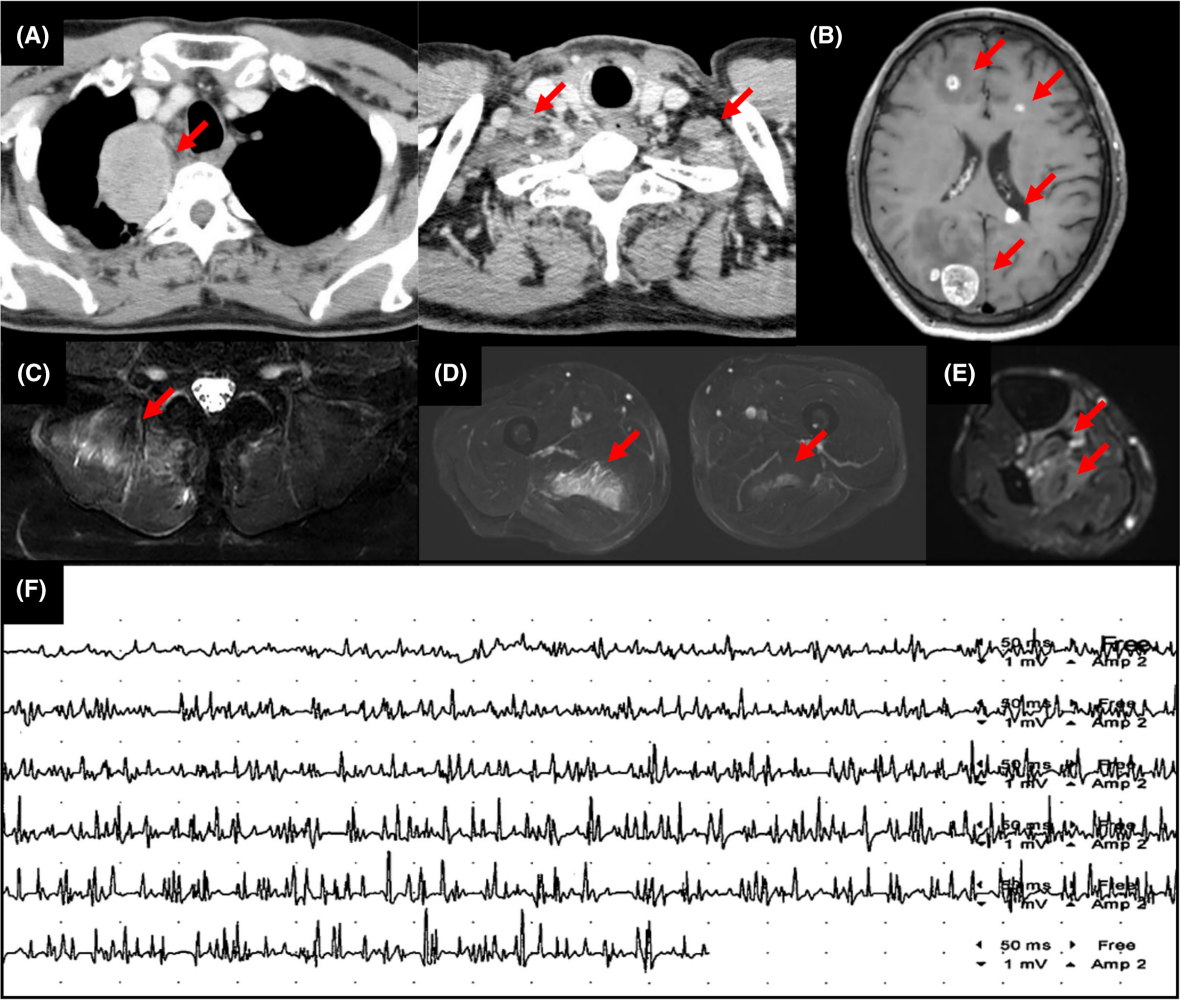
Diagnostic images of a 59‐year‐old male patient with lung adenocarcinoma. (A) Contrast‐enhanced computed tomography image shows lung cancer in the right upper lobe and bilateral supraclavicular lymphadenopathy. (B) Magnetic resonance imaging reveals multiple brain metastases. Axial STIR images shows hyperintense signals in the (C) right erector spinae muscles, (D) bilateral adductor magnus muscle, (E) right posterior tibial muscle, flexor digitorum longus muscle, and flexor hallucis longus (arrows). (F) Electromyography shows early recruitment and polyphasic, short‐duration, low‐amplitude motor unit action potentials.
